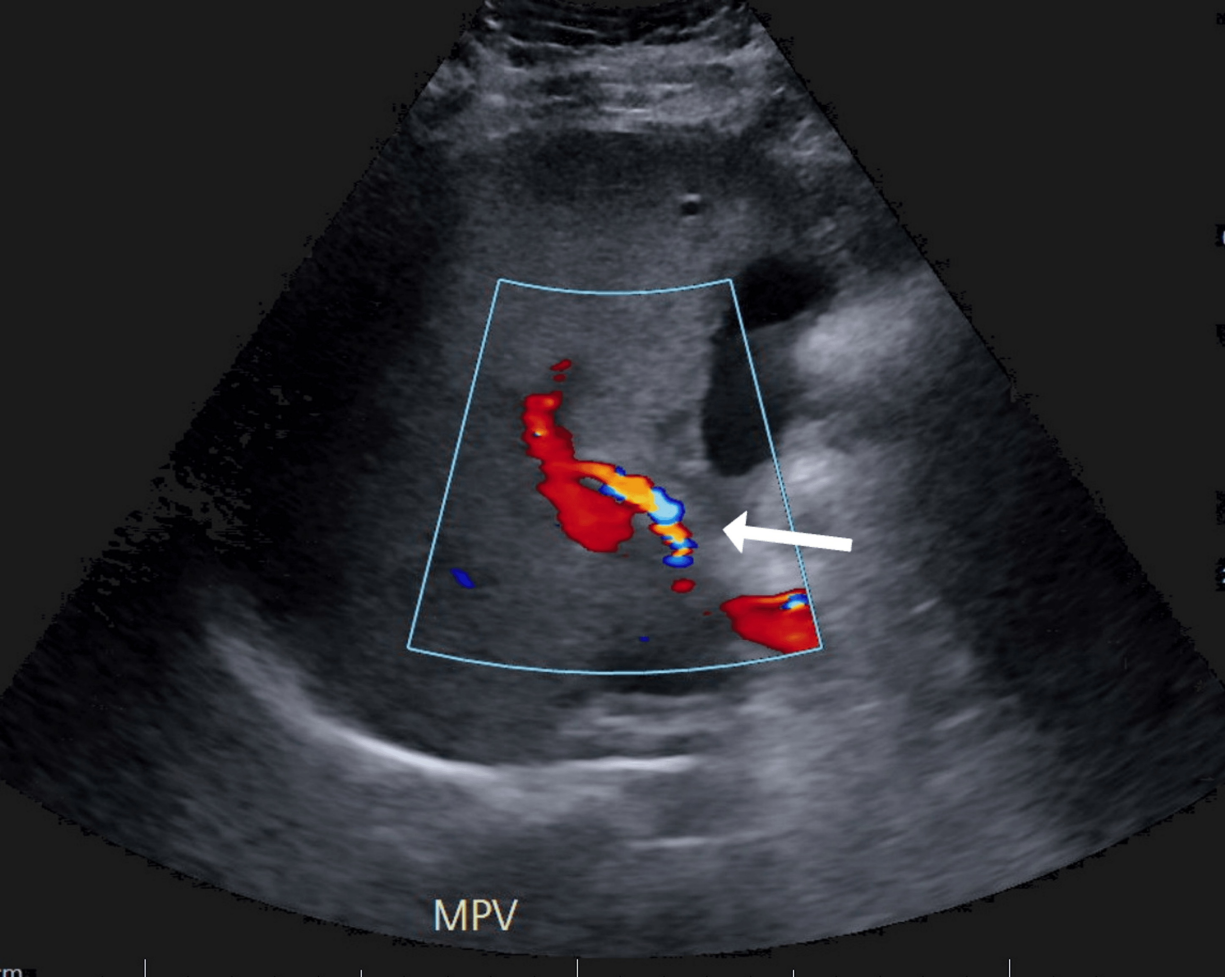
Doppler ultrasound showing blood flow in portal, hepatic and splenic veins (white arrow)
